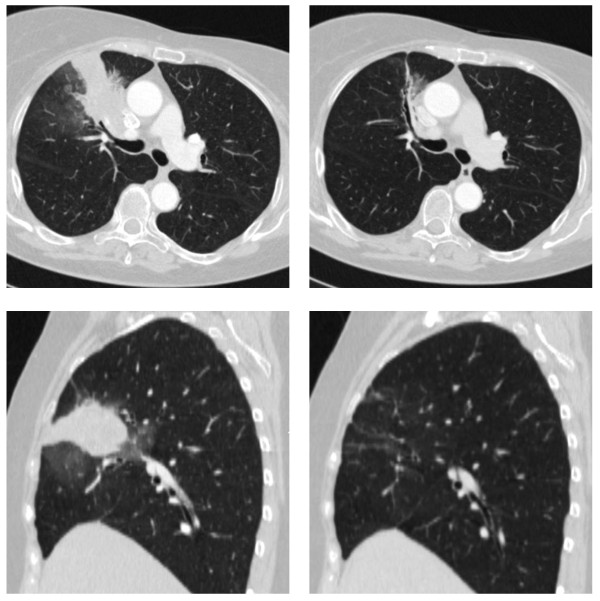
CT images of pre- and post-treatment for patient experiencing a complete response. Upper left: pre-treatment axial-mediastinal window. Upper right: post-treatment axial mediastinal window. Lower left: pre-treatment sagittal-mediastinal window. Lower right: post-treatment sagittal-mediastinal window.
